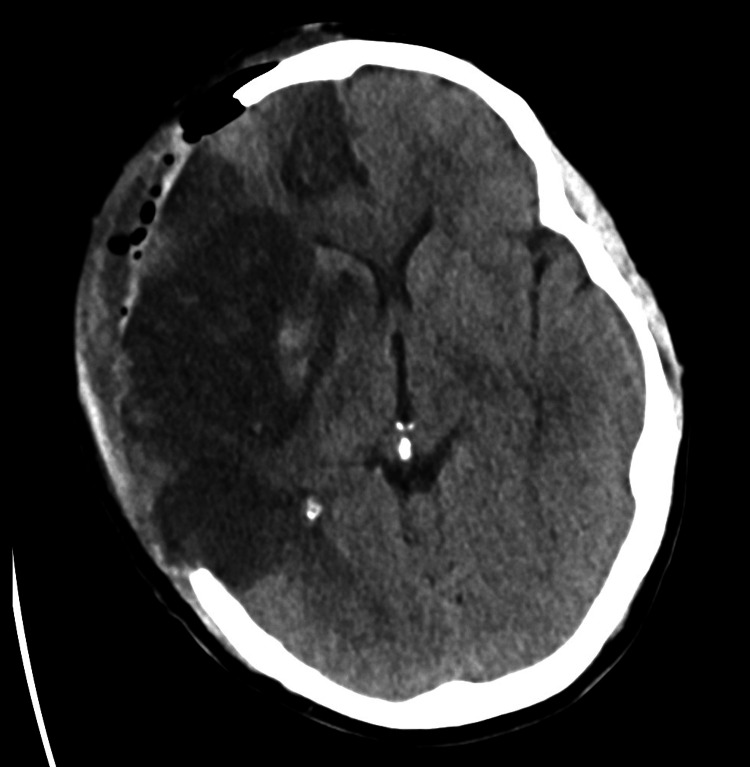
Post-craniectomy non-enhanced brain CT showing a large right middle cerebral artery infarct with resolving mass effect and no hemorrhagic transformation.
